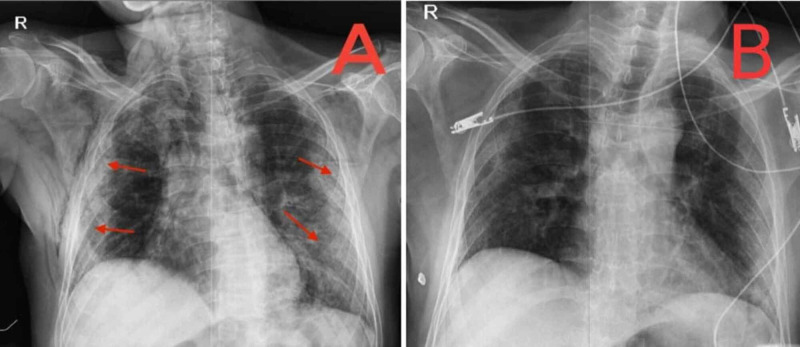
Chest X-ray of the patient Chest X-ray showing progression of bilateral peripheral and basal lung infiltrates on (A) the day of admission and (B) the day before discharge.
